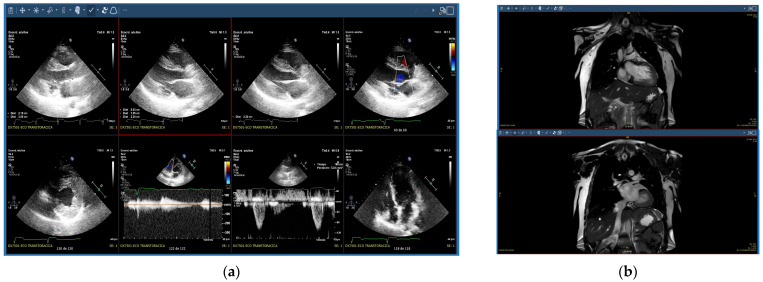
(a) Echocardiographic study in the affected daughter, (b) magnetic resonance imaging report of the affected daughter.
